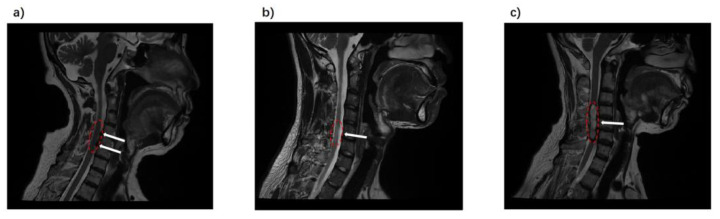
Examples of T2-weighted images of patients. (a) Cervical spondylosis. Disc herniation was seen at levels C4 to C6. (b) Cervical myelitis. High signal visible in C5/C6 segments. (c) Cervical tumors. The tumor lesion in the C4 to C6 segments was visible. Arrows and red border show the lesion location and segments.
